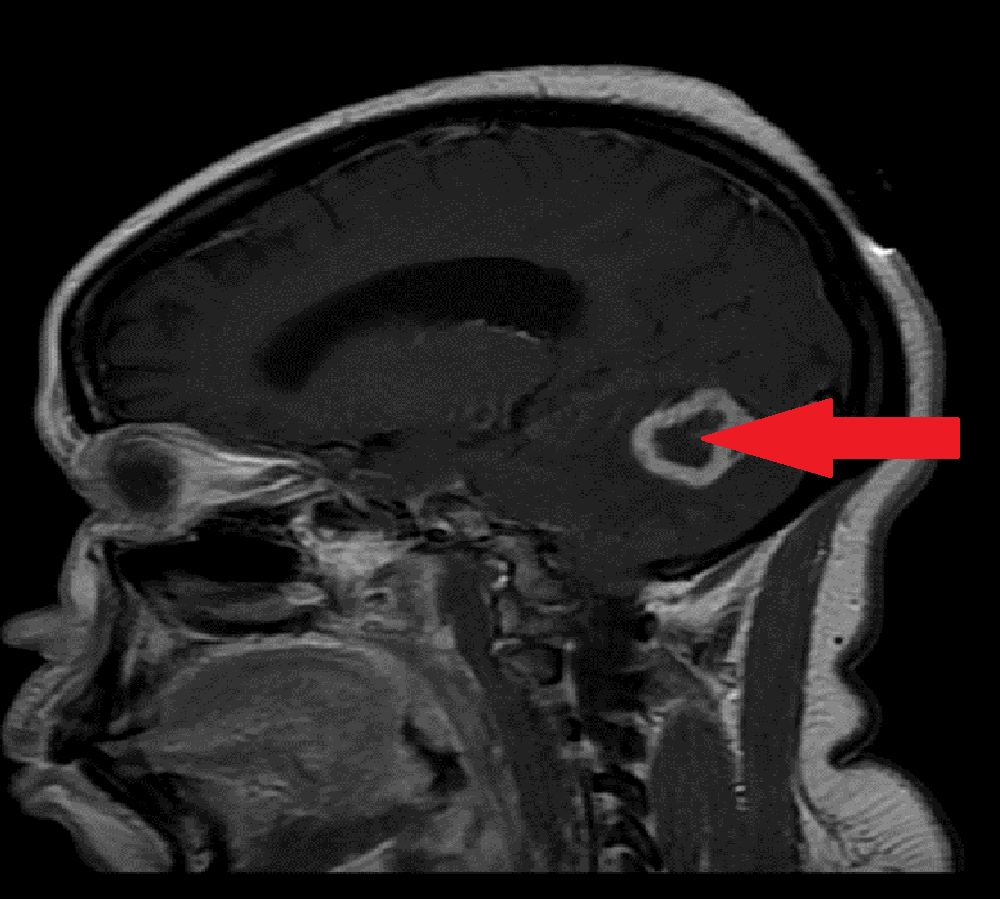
MRI of the brain (sagittal view) shows a large right cerebellar ring-enhancing lesion indicated by the arrow. MRI of the brain from the side (sagittal view) shows a large ring-enhancing mass in the cerebellum, which is seen as an irregular oval-appearing lesion with a bright white outline and is indicated by the arrow. MRI - magnetic resonance imaging.
